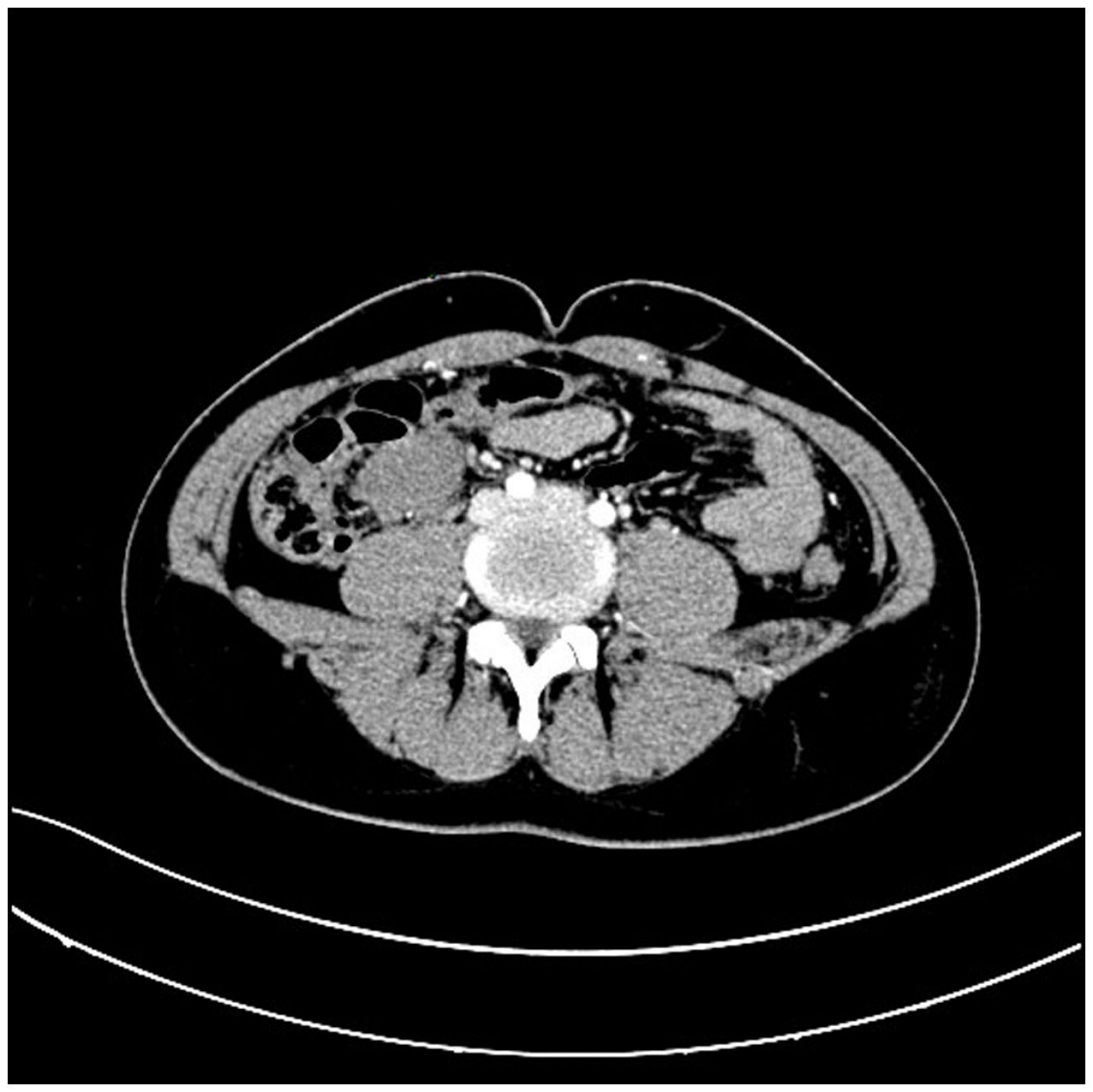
Abdominal CT scan revealed a well-defined mass in the right psoas muscle.
